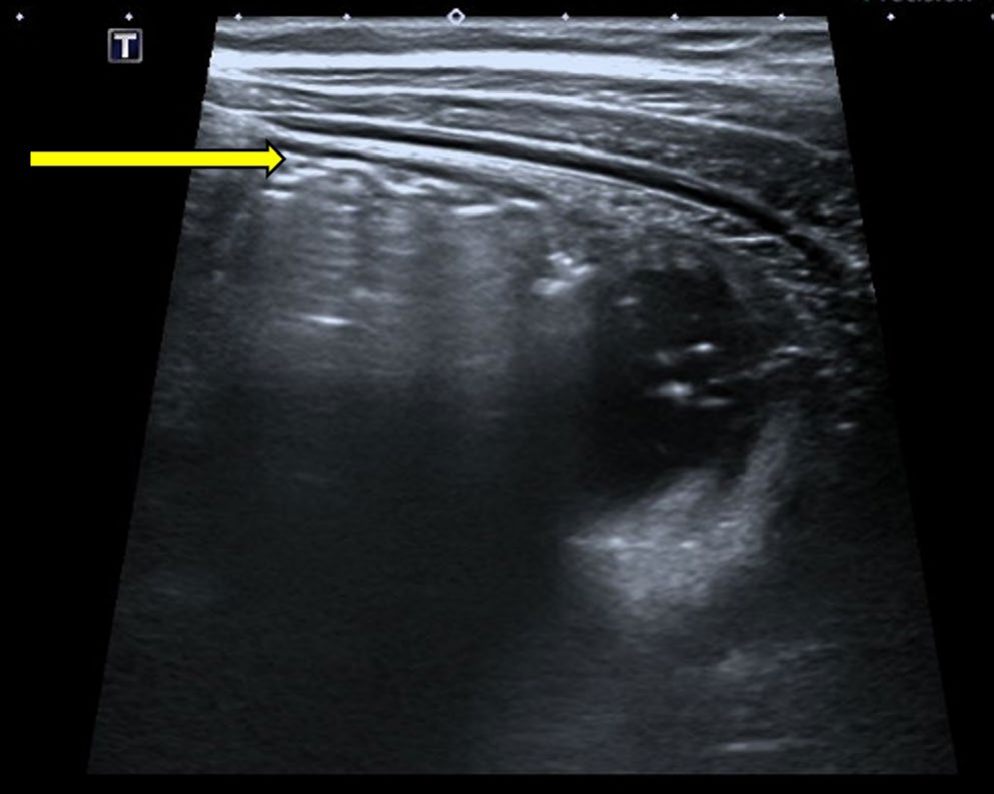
Abdominal ultrasound showing the presence of a mass associated with a superficial hyperechoic curvilinear structure (arrow) with posterior acoustic shadowing. This most likely represents a strand of hair within the trichobezoar
